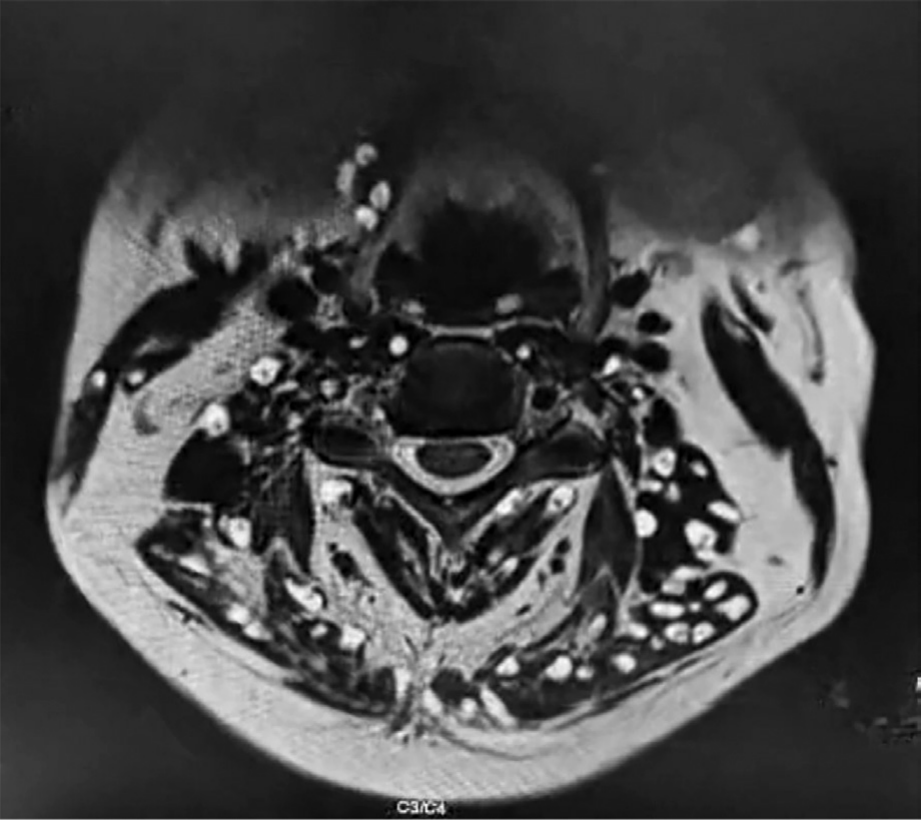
Description: Axial T2-weighted MRI at the C3–C4 vertebral level demonstrates multiple, well-defined hyperintense cystic lesions distributed within the paravertebral and posterior cervical musculature, many of these being with eccentric mural nodules representing the scolex. The cervical spinal cord and intervertebral discs are preserved without intramedullary signal changes. Findings are consistent with myocysticercosis involving cervical muscles. Clinical Significance: This axial view provides a cross-sectional perspective confirming muscular myocysticercosis with characteristic scolex identification. The preservation of spinal cord integrity and intervertebral disc morphology rules out compressive pathology. Differentiation from abscesses, neoplastic lesions, or inflammatory cysts is possible due to the presence of the scolex, which remains pathognomonic for cysticercosis. Origin: @GSVM Medical College, PMSSY Superspeciality Hospital, Kanpur, Uttar Pradesh, India. Pincode: 208002.
